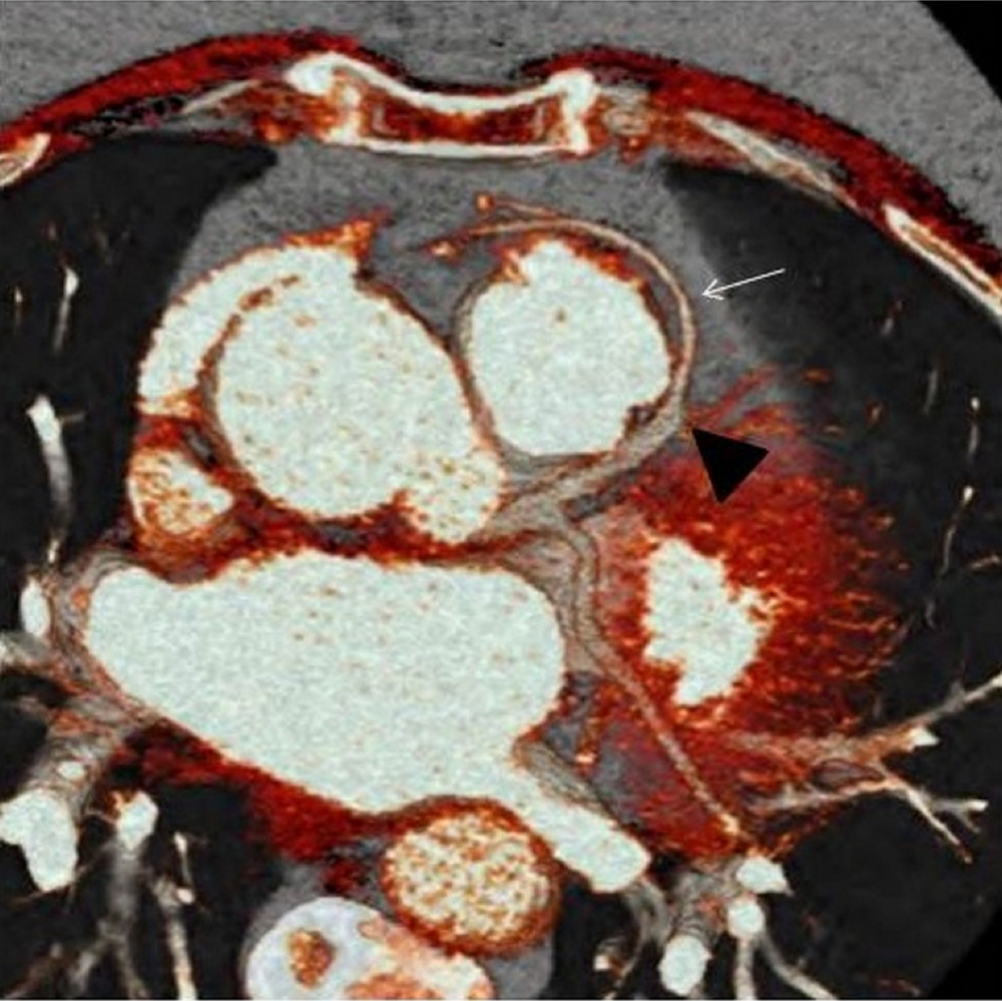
Cardiac CT image showing the left coronary artery and the aberrant RCA (small arrow) with its take-off from the mid-segment of the LAD. LAD, left anterior descending; RCA, right coronary artery.
